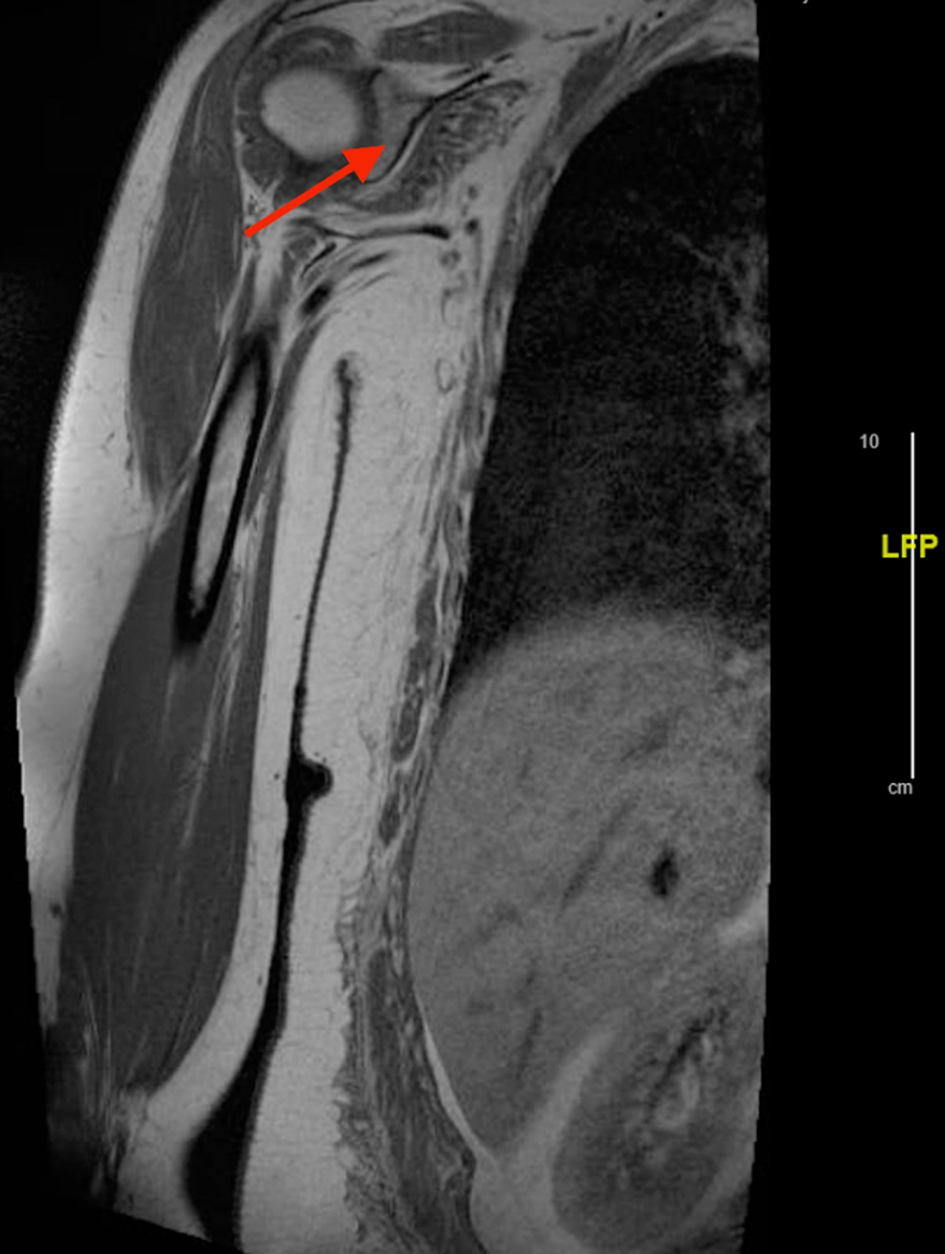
Coronal MRI of right shoulder Arrow demonstrates muscular edema of the shoulder girdle.
